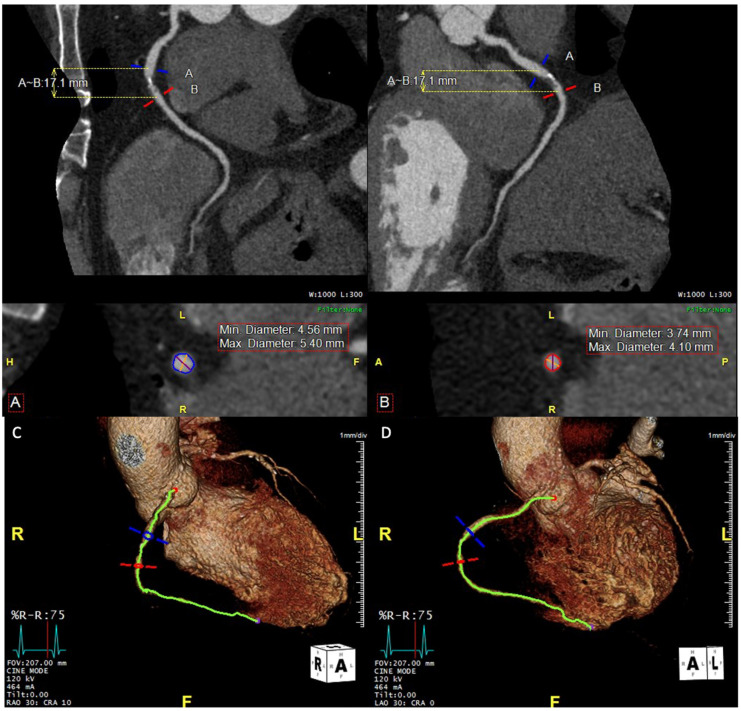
Coronary computed tomography angiography (CCTA) reveals severe stenosis in the mid-segment of the right coronary artery (RCA). (A) Red lines indicate the minimum diameter, and blue lines represent the maximum diameter of the proximal reference vessel. (B) Red lines show the minimum diameter, and blue lines indicate the maximum diameter of the distal reference vessel. A–B represents the lesion length. Volume-rendered CCTA images with fluoroscopic angles showing RCA stenosis: (C) RAO 30°, CRA 10°; (D) LAO 30°, CRA 0°. RAO: right anterior oblique; CRA: cranial; LAO: left anterior oblique.
